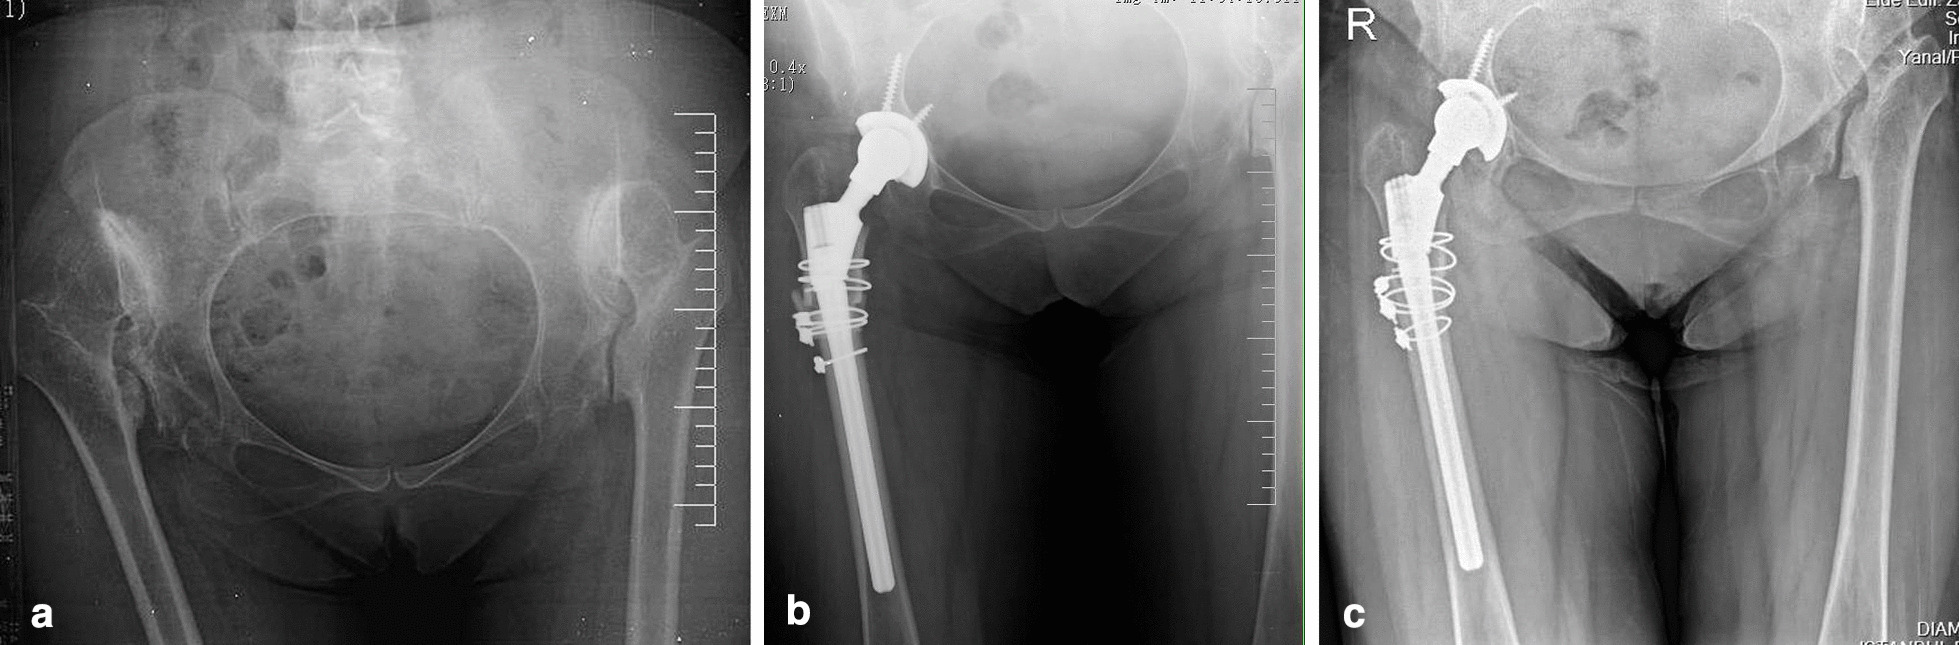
Radiographs of a 54 years old female with high hip dislocation. a Preoperative anteroposterior view. b Early postoperative radiographic image. Hip was reconstructed at the level of the anatomic hip center by cementless modular (Helios, Valencia, Biomet, Spain) total hip arthroplasty combined with transverse osteotomy and the osteotomy site was wrapped with the osteotomy fragments, stabilized using cerclage wires. Reconstruction of the acetabulum with screws was performed. c Postoperative radiographic image at 16-month follow-up, bone union was detected at the osteotomy site and no radiolucent lines around the femoral stem were found
